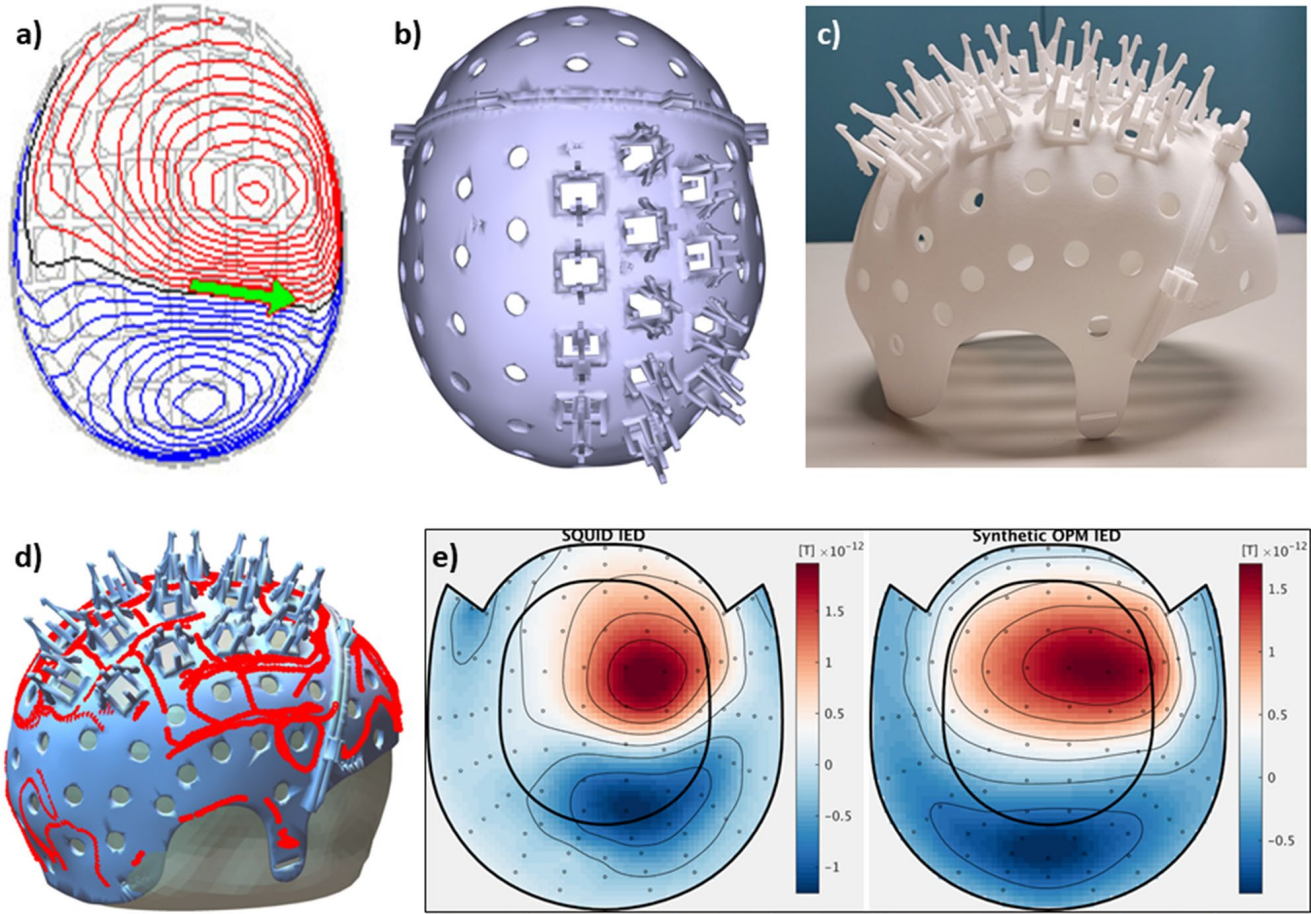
Helmet design for patient #4 and field patterns recorded with SQUIDs and OPMs. (a) Field pattern produced by IED (green arrow indicates the source-reconstructed equivalent current dipole) in the previously recorded clinical MEG, originating from, and in agreement with, a right central focal cortical dysplasia. (b) 3D-helmet model, including removable front and OPM-holders. (c) 3D-printed helmet. (d) Digitised helmet points (red dots) aligned with helmet-model, and co-registered to the anatomy (head surface from MRI). (e) Magnetometer field pattern for an IED (at the time point of maximum SNR) recorded with the SQUID-based system (left), as well as field pattern for an IED recorded with the OPMs, projected onto the SQUID-sensor layout (using inverse/forward projection with minimum norm^52,53^). Note the good agreement between the IED field patterns, despite the limited sampling with the OPMs, suggesting that both systems recorded similar phenomena. Also note the agreement with the previously recorded IED (panel a) (see also Fig. S5).
